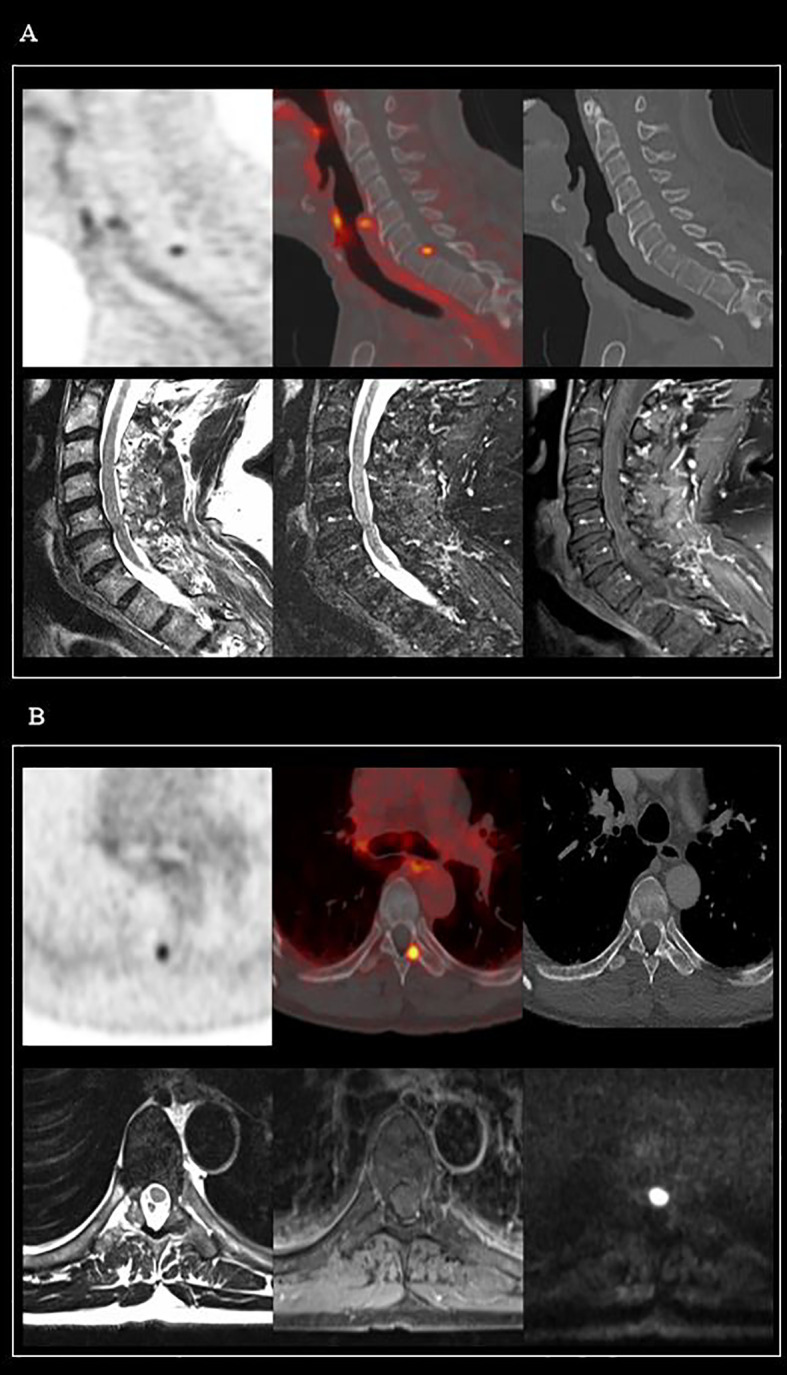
Two cases of PSMA-avid bone uptakes, with no evidence of metastatic lesions on MRI imaging. (A)
